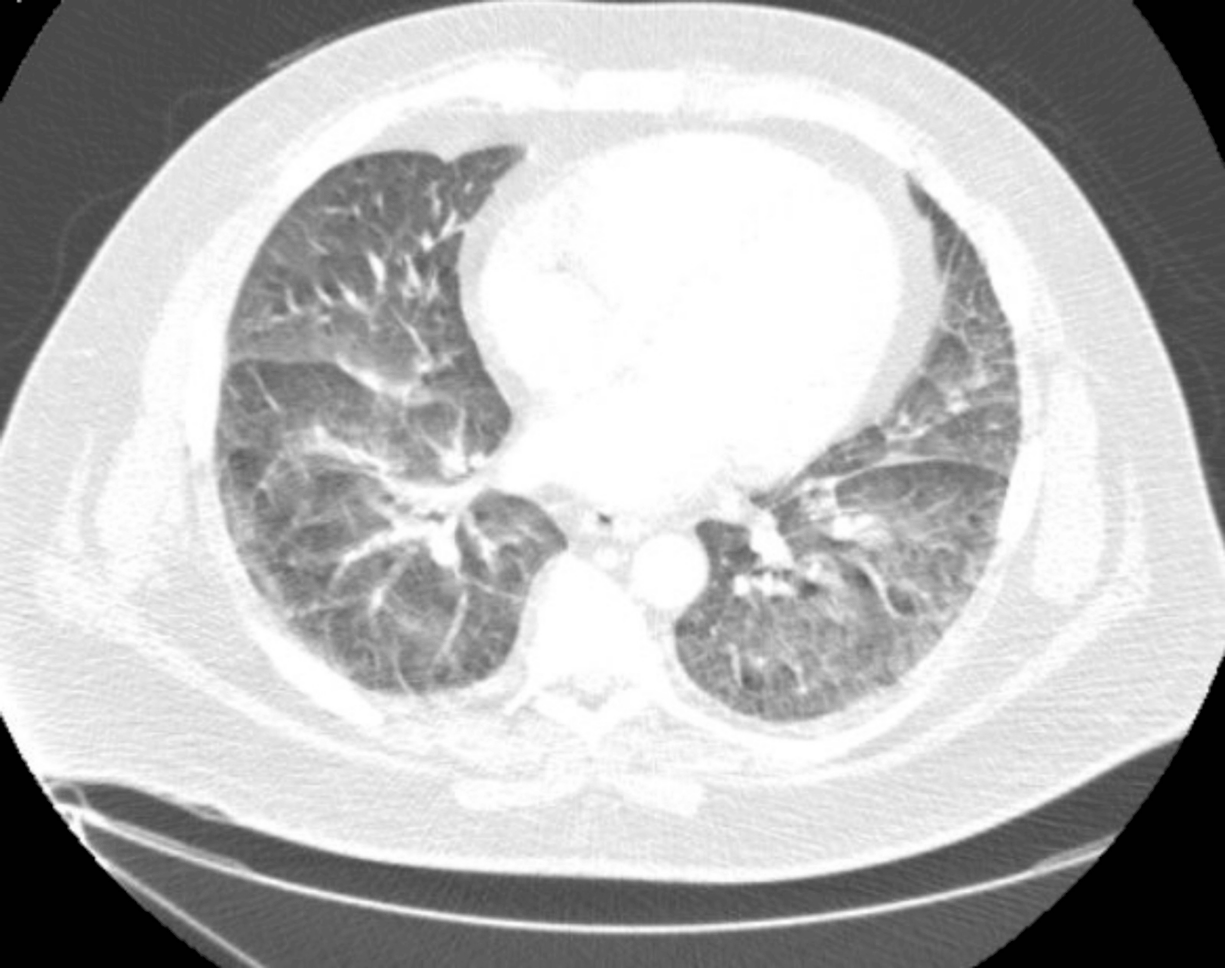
CT angiogram of chest showing diffuse pulmonary edema, ground glass opacities, mild bibasilar pleural effusions, and cardiomegaly.
